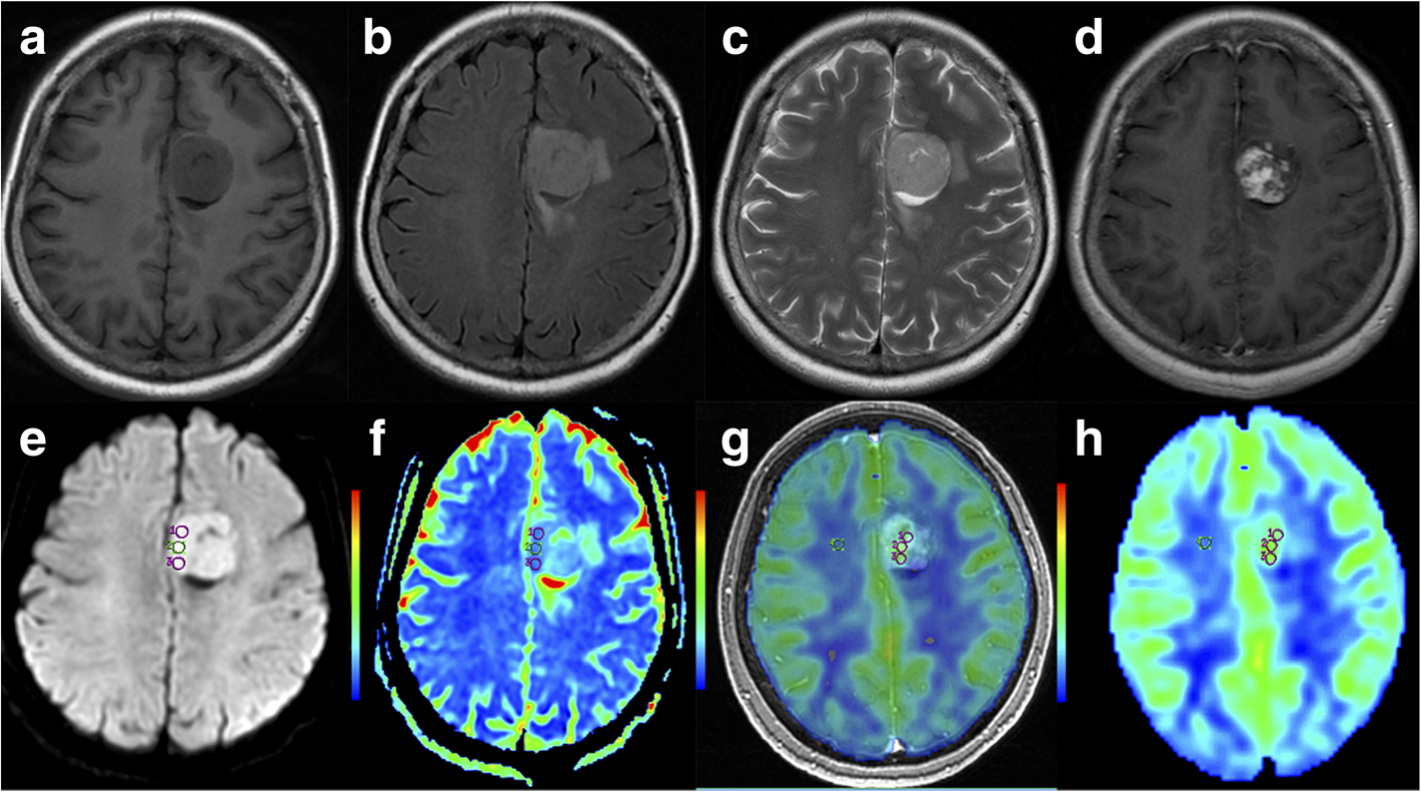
Structural (a, b, c and d) and advanced (e, f, g and h) MR images obtained from a 53-year-old female showing left frontal lobe GBM with MGMT promoter methylation. a Precontrast T_1_-weighted image. b Fluid-attenuated inversion recovery image. c T_2_-weighted image. d Postcontrast T_1_-weighted image. e DWI map. f ADC map: the mean ADC value of the three small ROI was 0.722 × 10^− 3^ mm^2^/s. g The map fused CBF and 3D–T1WI/C. h CBF map: the mean rCBF of the three small ROI was 3.94
